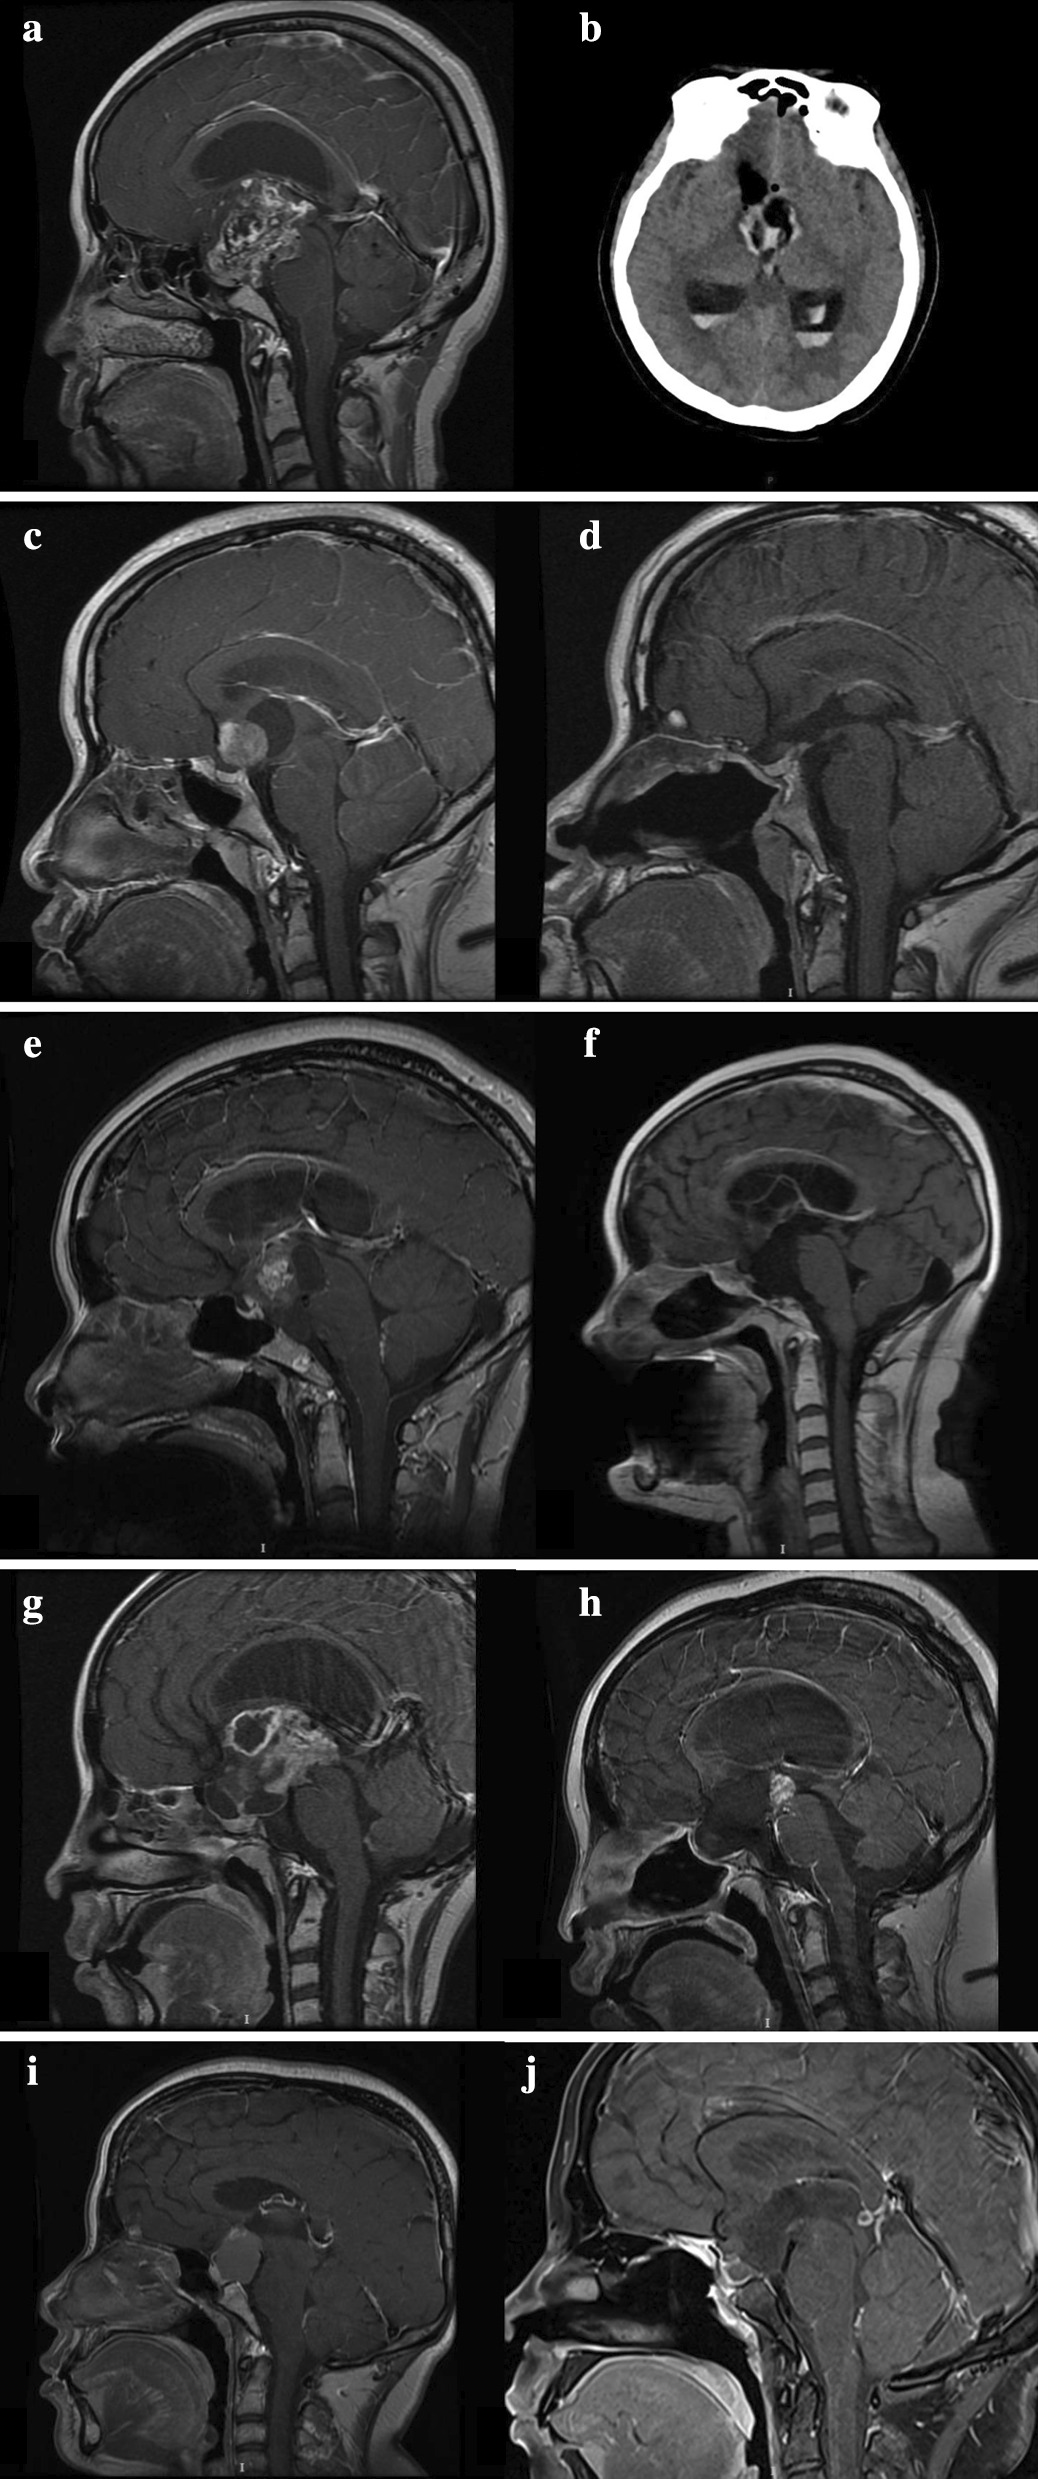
Comparison of the sagittal MRI obtained at pre- and post-operation. a, b: Case 1. The preoperative MRI shows a giant hypothalamic tumor with no clear margin between the tumor and hypothalamic structure. The postoperative CT (after one day) shows the GTR of the tumor and hematocele in the lateral ventricles. c, d: Case 2. The preoperative MRI study shows the suprasellar tumor with solid and cystic portions. The postoperative MRI study obtained at one year after surgery shows that the gland and the stalk were preserved and visible. The tumor was radically resected. e, f: Case 3. The preoperative MRI shows the tumor located suprasellar, interpeduncular and prepontine cistern. The postoperative MRI (6 months) shows the radical resection of the hypothalamic glioma. g, h: Case 4. The preoperative and postoperative (one year) MRI shows the suprasellar hypothalamus glioma with the involvement of the third ventricle. The residual tumor remained stable after the subtotal resection. i, j: Case 5. The preoperative and postoperative (6 months) MRI shows the radical resection of the hypothalamic glioma
